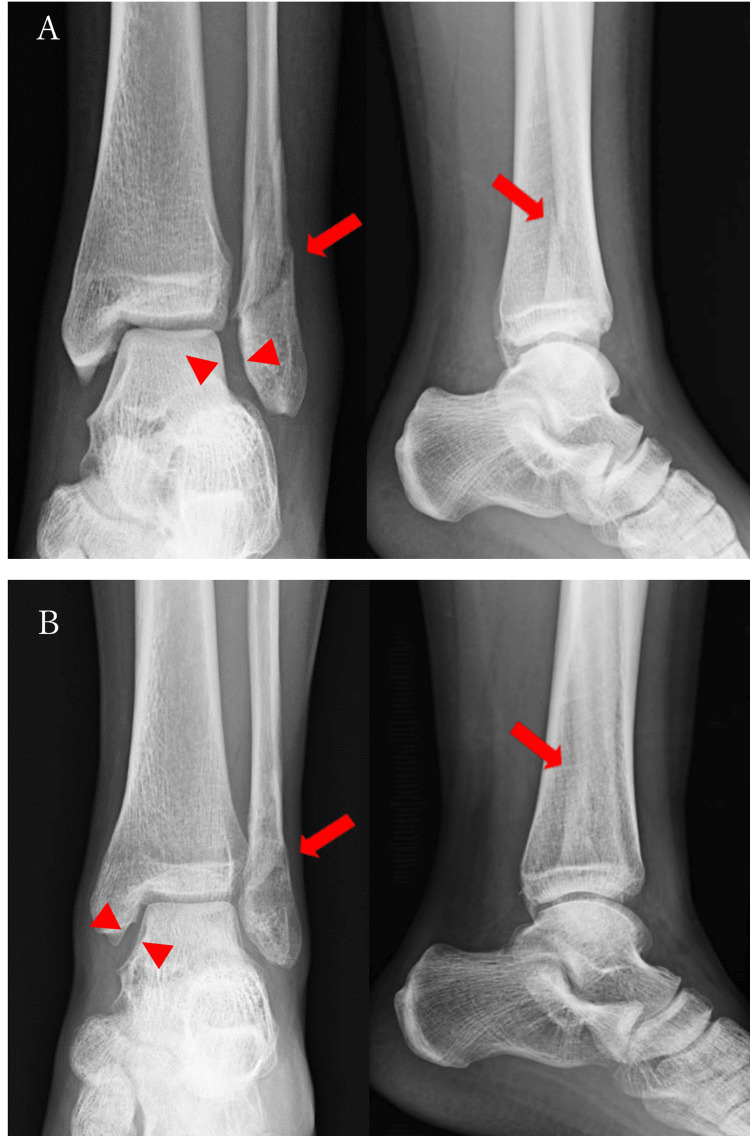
Radiographs of the patient (A) Plain X-ray frontal and lateral views obtained at the time of injury showed that the distal end of the fibula was obliquely fractured, displaced posterior-laterally, and 2.5 mm shorter (arrow), and the lateral joint space was dilated (arrowhead). (B) Plain X-ray frontal and lateral images obtained at the time of arrival showed mild enlargement of the medial joint space (arrowhead).
